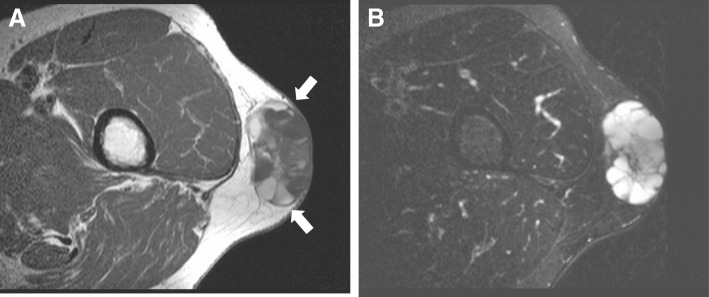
Magnetic resonance imaging showed a well-circumscribed multilocular cystic mass approximately 5 cm in diameter. The mass (*arrows*) comprised T1 low-signal (a) and T2 high-signal (b) intensity compartments
